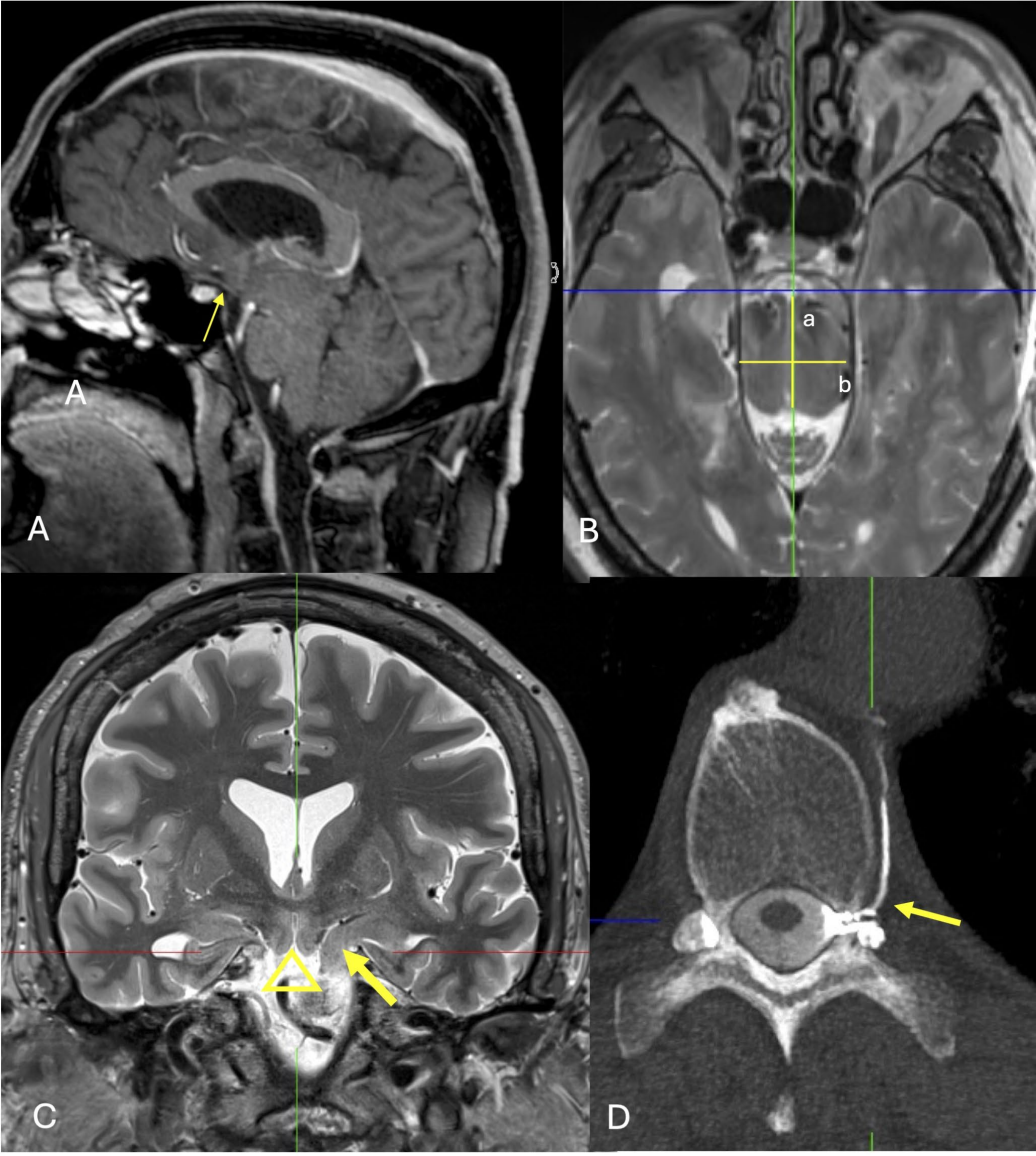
Sagittal contrast-enhanced MPRAGE (A), axial T2-weighted SPACE (B), coronal T2-weighted STIR images (C) in a 56-year-old man with apathy since four years. Note the sucking of the brainstem, of the mesial temporal lobes, of the basal frontal lobes towards the interpeduncular cistern. The optic tract is sucked downwards (A: arrow), the upper brainstem has a longer a.p. diameter (B: a/b), the left hippocampus is sucked over the petroclinoid fold (C: arrow), and the basal frontal lobes point downwards (C: triangle). Dynamic left lateral CT myelography revealed a left-sided CSF venous fistula at Th10/11 (D: arrow). The patient rapidly improved after surgical ligation
